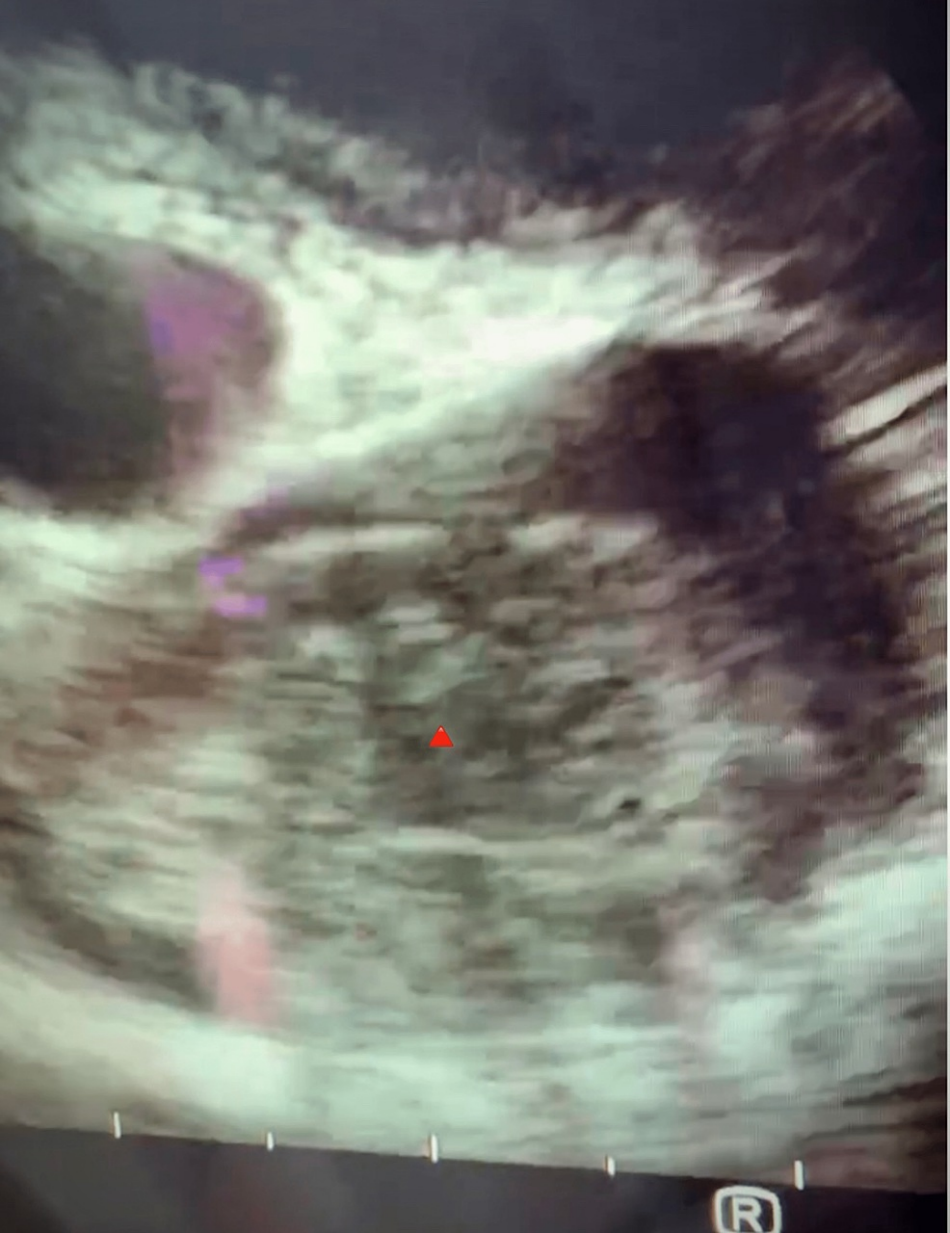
An intraluminal tumor thrombus (red triangle) within the SVC diagnosed by Endobronchial Ultrasound with Trans-bronchial Needle Aspiration (EBUS-TBNA). The patent lumen, posterior to the SVC, is the aorta. SVC- superior vena cava;
